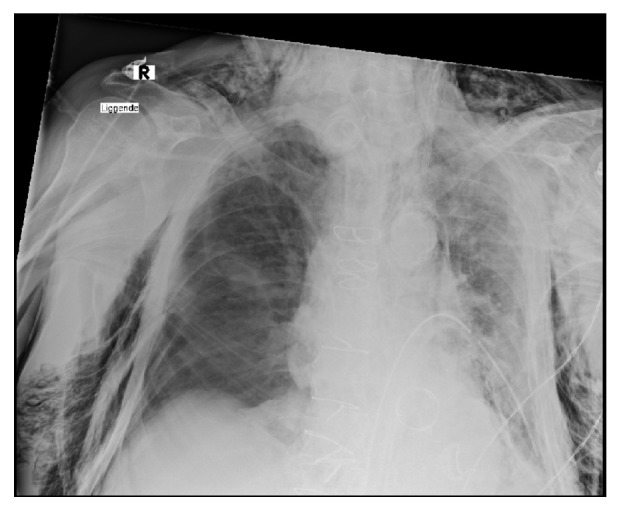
Chest X-ray is showing massive subcutaneous emphysema after CICO and reestablishing a surgical airway.
